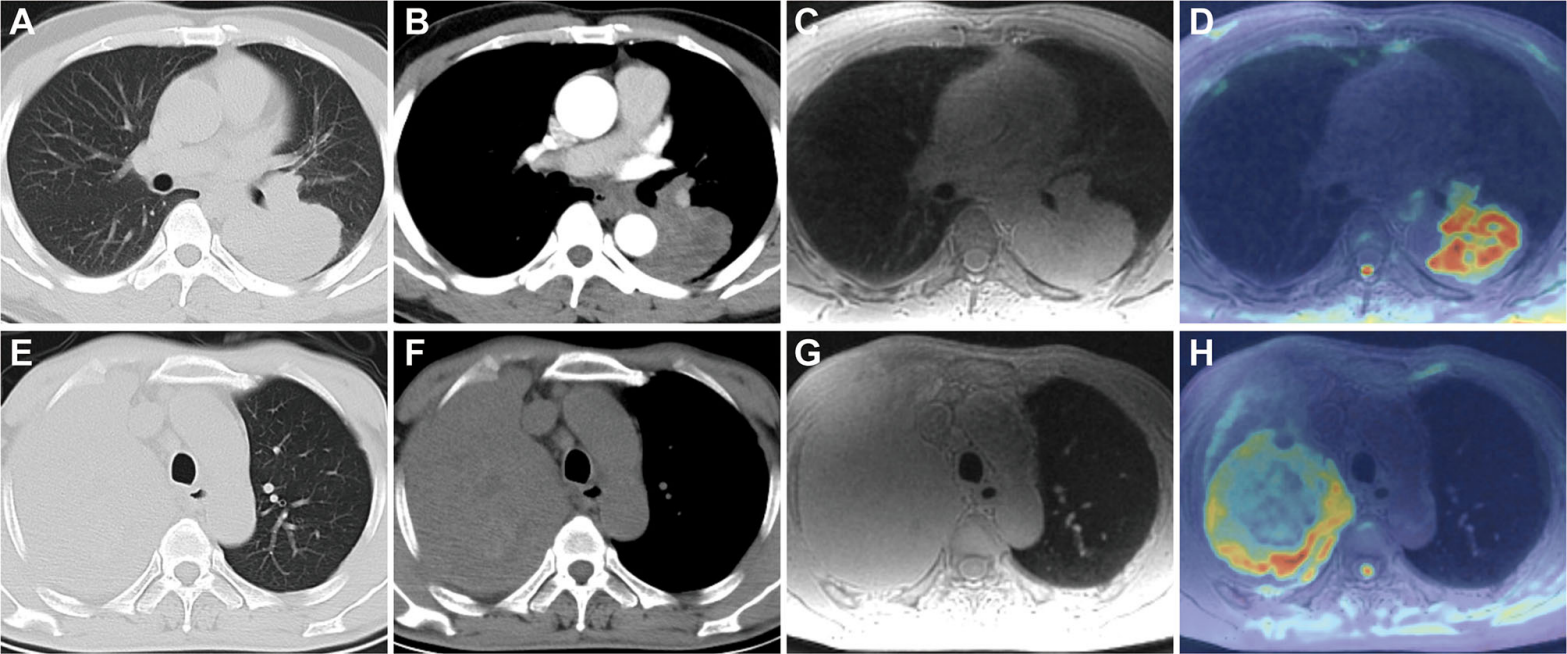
Lung CT and MRI images of two patients without or with significant inter-modality variation of volume. A 48-year-old man with lung adenocarcinoma in the left lower lobe on axial CT lung window (A), mediastinal window (B), and ZTE-MRI (C); ADC map (D) showed the consistent lesion extent with ZTE-MRI and CT; a 59-year-old man with lung adenocarcinoma in the right upper lobe on axial CT lung window (E), mediastinal window (F), and ZTE-MRI (G); ADC map (H) shows the actual lesion extent which was inconsistent with ZTE-MRI; it is confusing to distinguish the boundary between the non-smooth lesion and surrounding lung tissue with obstructive atelectasis on ZTE-MRI.
